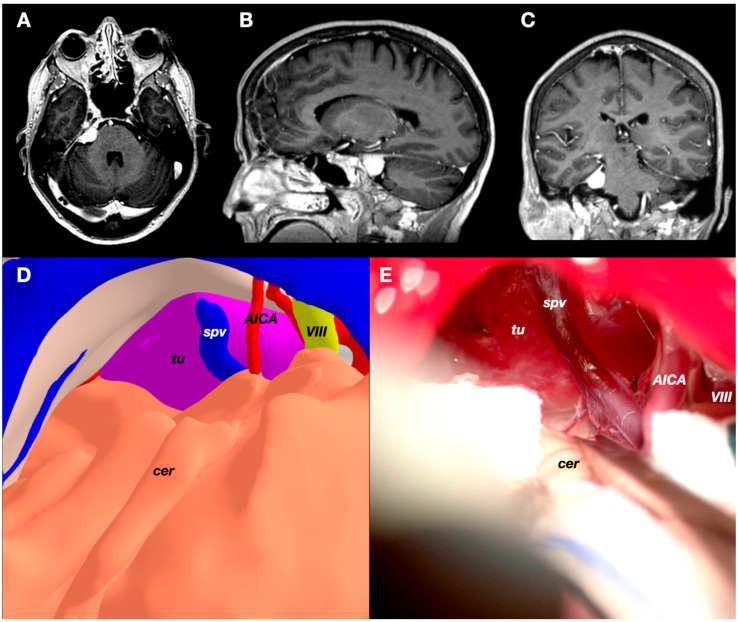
(A–C) Preoperative T1-GD-weighted MRI sequences of Case 3 showing a petrous apex meningioma on the right side. (D) VR 3D view where the cerebellum (cer), tumor (tu), superior petrosal vein (spv), VIII cranial nerve (VIII), and a distal branch of AICA (AICA) are shown. (E) Intraoperative view of the petrous apex meningioma showing the same neurovascular structures.
